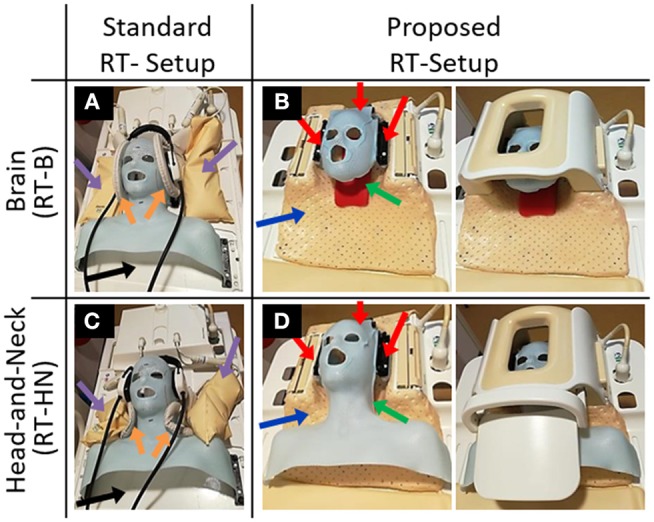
Standard and proposed setups for MR imaging in treatment position for brain (A,B) and head-and-neck (C,D) radiotherapy patients. The blue arrows indicate the newly designed thermoplastic holder. The red arrows indicate the anchor points of the immobilization masks. The green arrows indicate the neck support, which can be made patient-specific.
